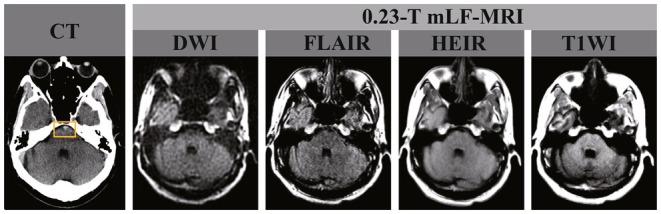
Special case of subarachnoid hemorrhage detected by CT but not visualized on 0.23 T mLF‐MRI. Case presentation: The patient presented with acute headache without focal neurological deficits. CT performed approximately 50 h after symptom onset demonstrated subarachnoid hemorrhage, and mLF‐MRI was subsequently completed approximately 19 h after the CT examination. CT, computed tomography; DWI, diffusion‐weighted imaging; FLAIR, fluid‐attenuated inversion recovery; HEIR, hematoma enhanced inversion recovery; mLF‐MRI, mobile low‐field magnetic resonance imaging; T1WI, T1‐weighted imaging.
